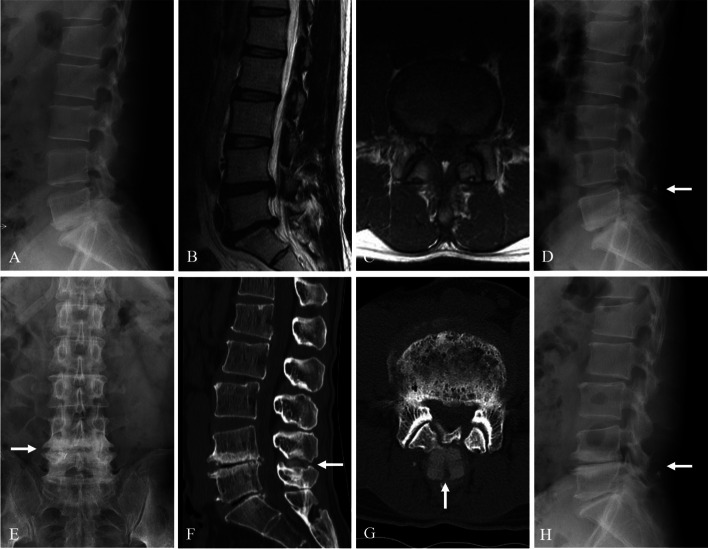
A 38-year-old man with lumbar disc herniation (LDH) at L4–5 and L5–6. Lateral (A) radiograph showing mild degenerative changes at L4–5 and L5–6. Magnetic resonance images (B and C) showed LDH at L4–5 and L5–6. The patient underwent discectomy and DIAM implantation at L4–5 (white arrow) (D). At 15 years after discectomy at L4–5 and L5–6 and DIAM implantation at L4–5, anteroposterior radiograph (E and H) and computed tomography scans (F and G) show severe degenerative changes at L4–5 and L5–6 and maintenance of DIAM at L4–5 (white arrows)
